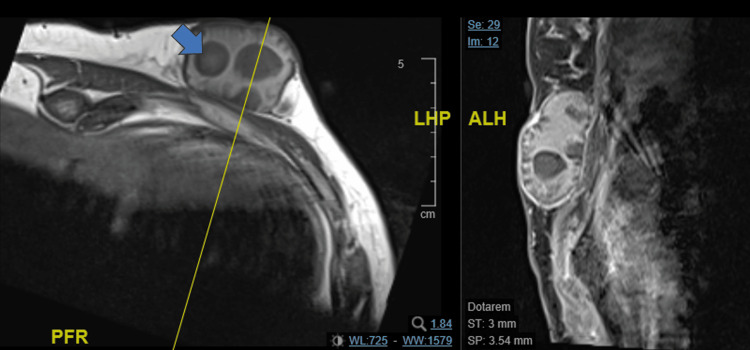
Axial and sagittal T1 weighted contrast MRI images of the lesion The solid component is depicted by an arrow with surrounding fluid MRI, magnetic resonance imaging
